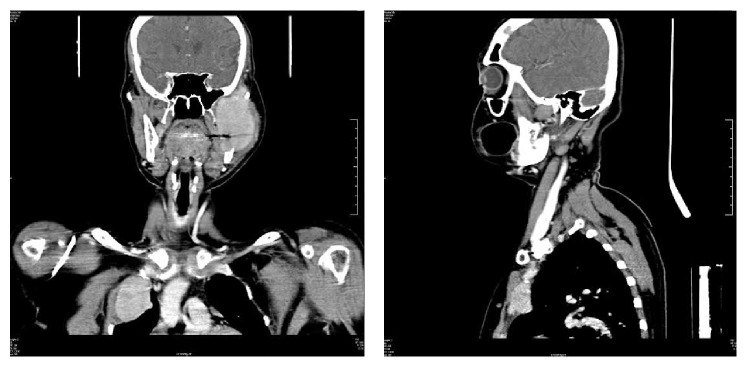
Coronal and sagittal sections of CT demonstrating the extent of the lesion.
